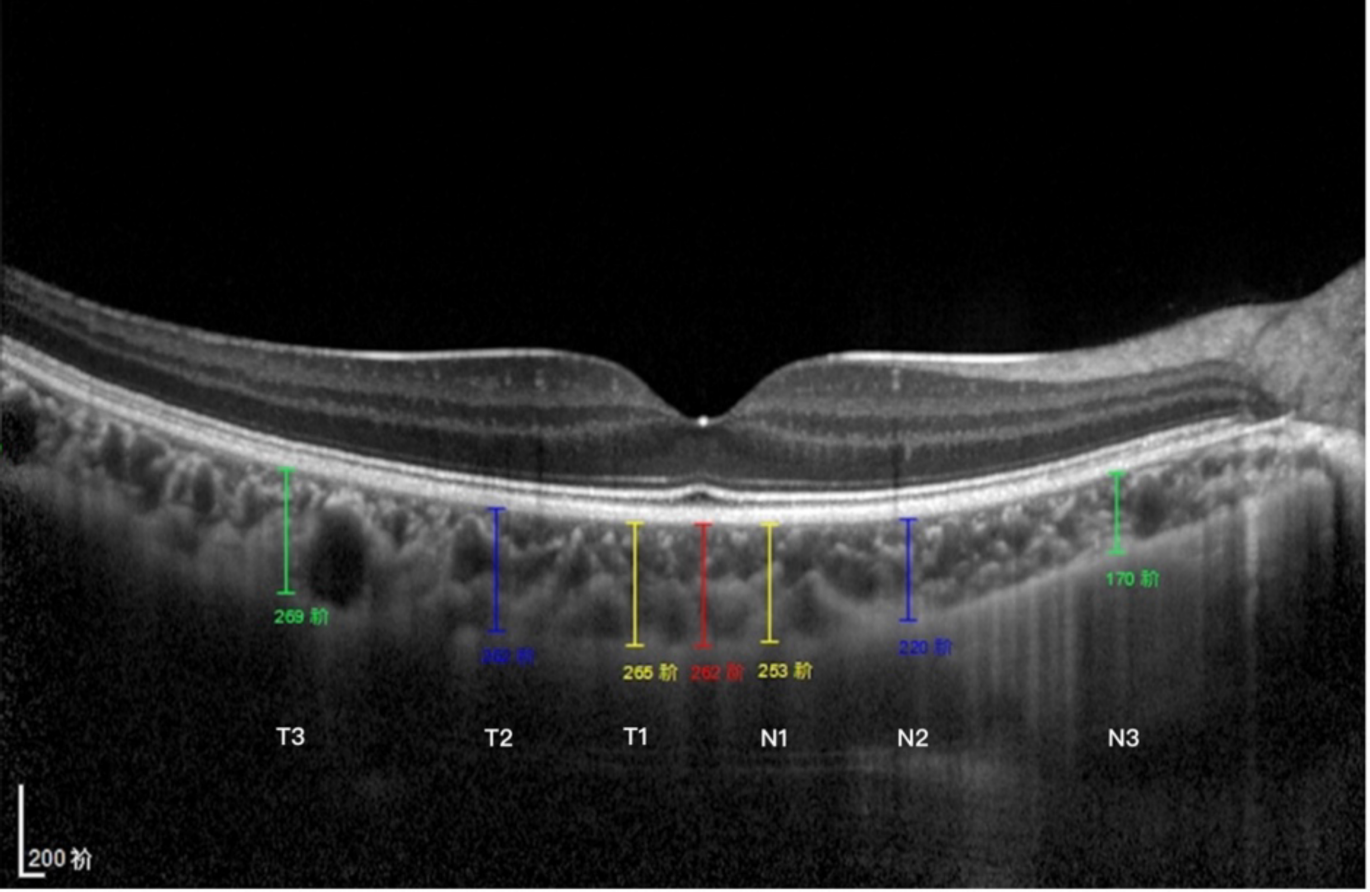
Subfoveal CT measurement. CT was measured at 7 points: directly beneath the fovea or the subfoveal area (SFCT) point, and 0.5/1.5/3 mm to the fovea nasally (N1/N2/N3), 0.5/1.5/3 mm to the fovea temporally (T1/T2/T3).
